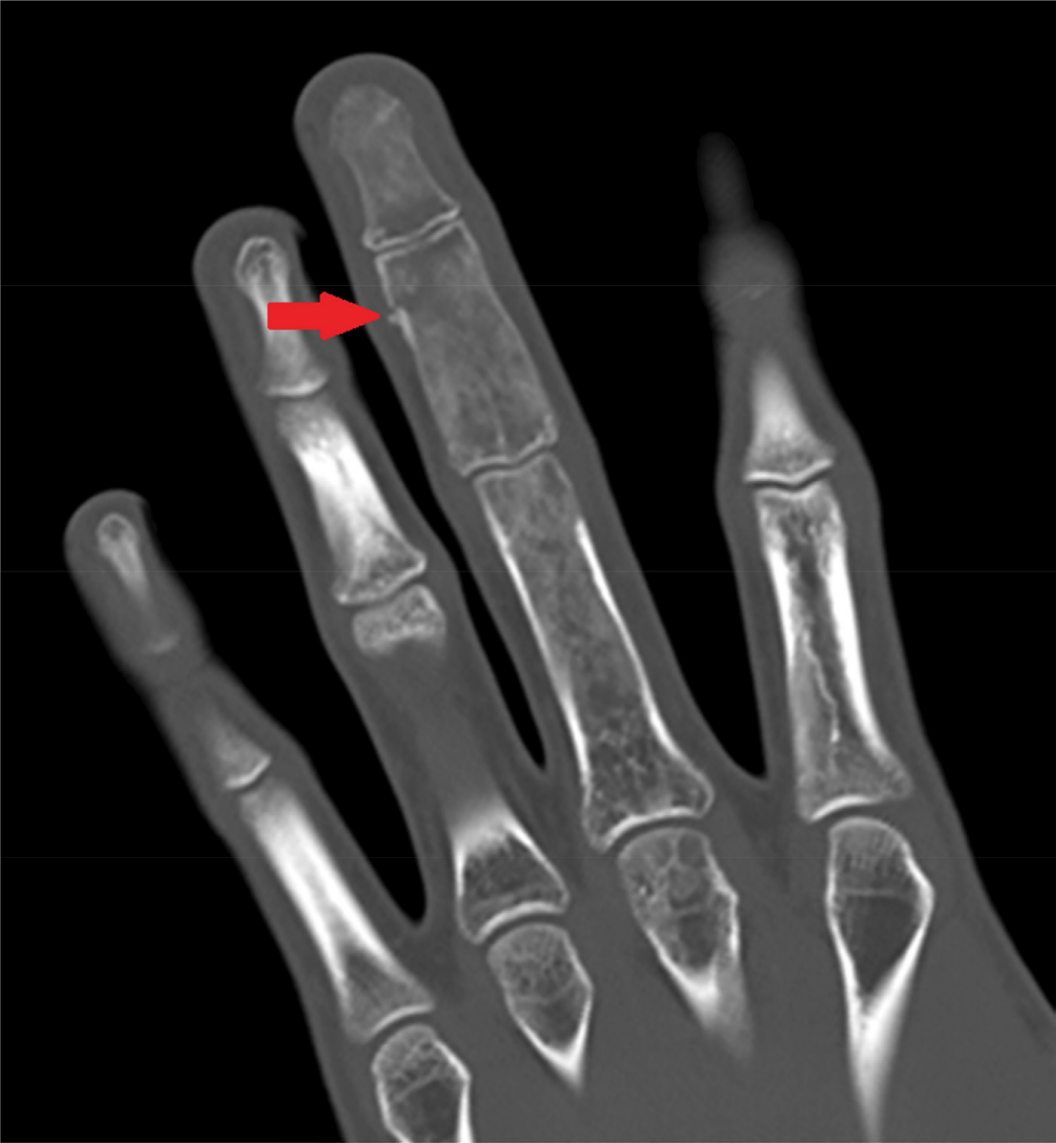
CT scan of the left hand showing multiple ground-glass bone lesions and a cortical fracture of the middle phalanx.
